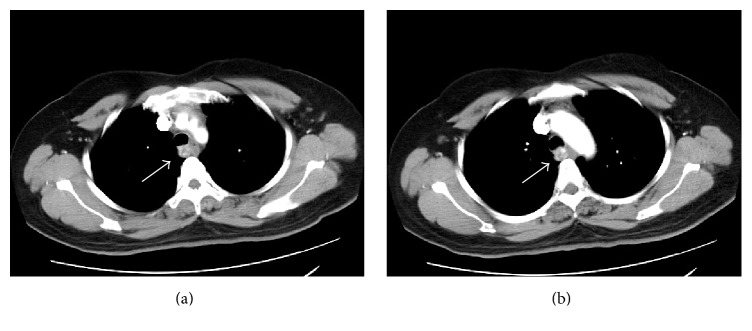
Thoracic computed tomography image showing an intensely enhanced lesion in the posterior superior mediastinum within the prevascular space.
